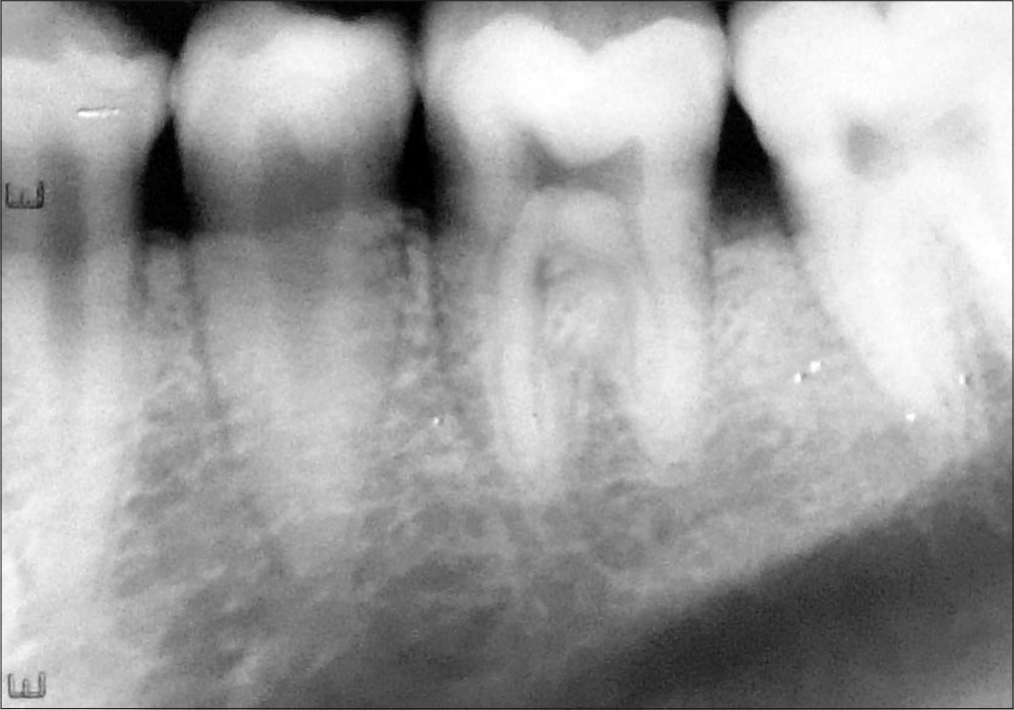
Postop radiograph (left side)
